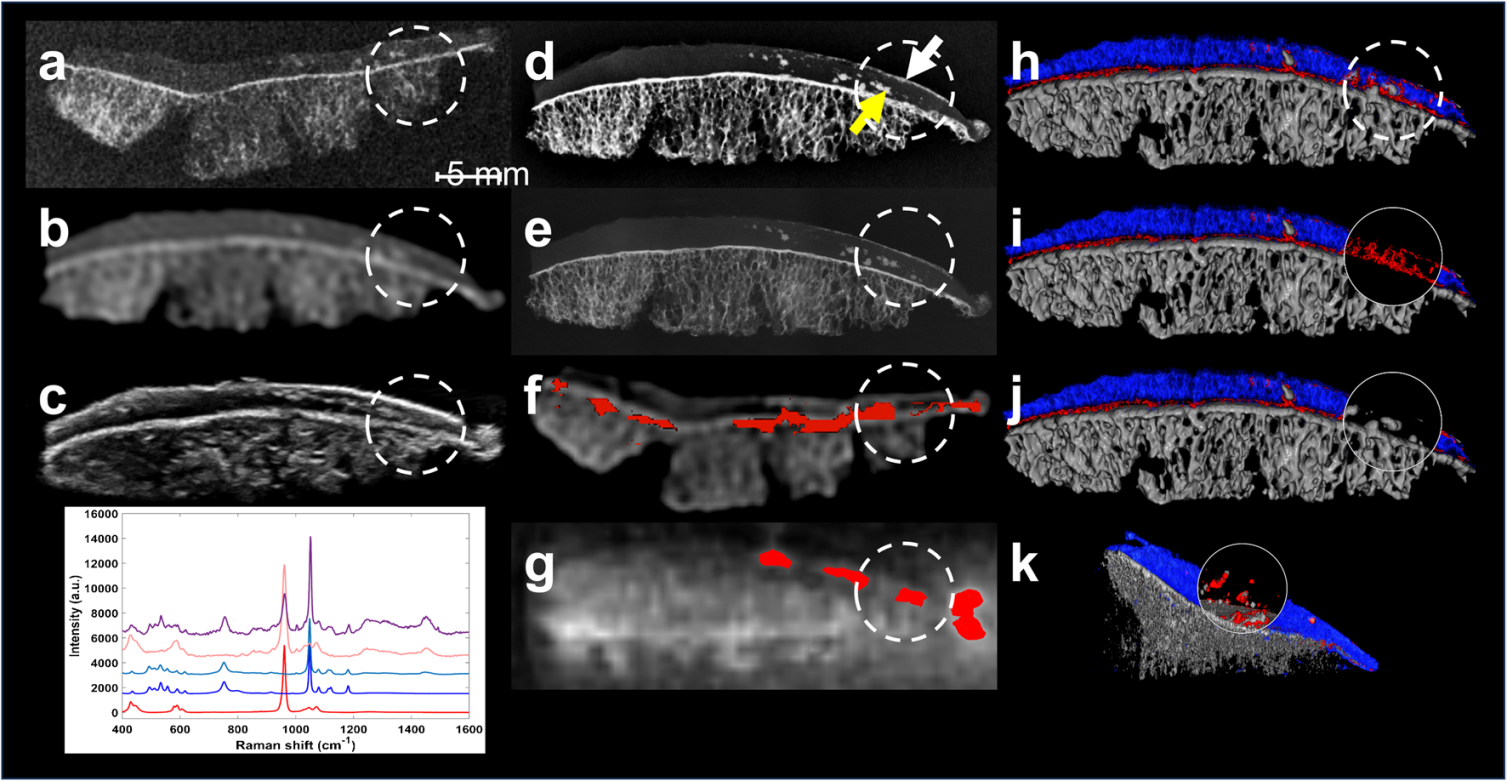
Detection and characterization of crystal deposits. a Digital radiography, b conventional CT, c ultrasound, d digital mammography, e micro-CT, f dual-energy CT (DECT), g ultrashort echo time (UTE) MRI, h–k multi-energy photon-counting-detector CT (PCD-CT). In UTE MRI, the articular cartilage calcium crystal deposition as a whole is color-coded in red due to its inability to discriminate between CPP and HA crystal deposits; in PCD-CT, the articular cartilage water content is color-coded in blue. While all conventional and advanced clinical imaging techniques were able to detect calcium crystal deposition within the articular cartilage (yellow arrow, dashed circle)—with varying levels of accuracy and sharpness/blur mainly due to spatial resolution differences—DECT and UTE MRI both failed to identify crystal deposits on the cartilage surface (white arrow, dashed circle). Additionally, although DECT, UTE MRI, and PCD-CT were all able to quantify calcium crystal deposition with varying accuracy, PCD-CT was the sole technique capable of distinguishing between CPP (color-coded in red) and HA (color-coded in white) crystal aggregates. (l) Raman spectroscopy was used as the reference standard (synthetic CPP and HA spectra are color-coded in blue and red, respectively) and confirmed the co- existence of CPP and HA crystals in two of the three cartilage scrapings/biopsies from the tibial plateau sample. Reprinted with permission from Bernabei et al. Multi-energy photon-counting computed tomography versus other clinical imaging techniques for the identification of articular calcium crystal deposition. Rheumatology (Oxford). 2021;60:2483–2485
